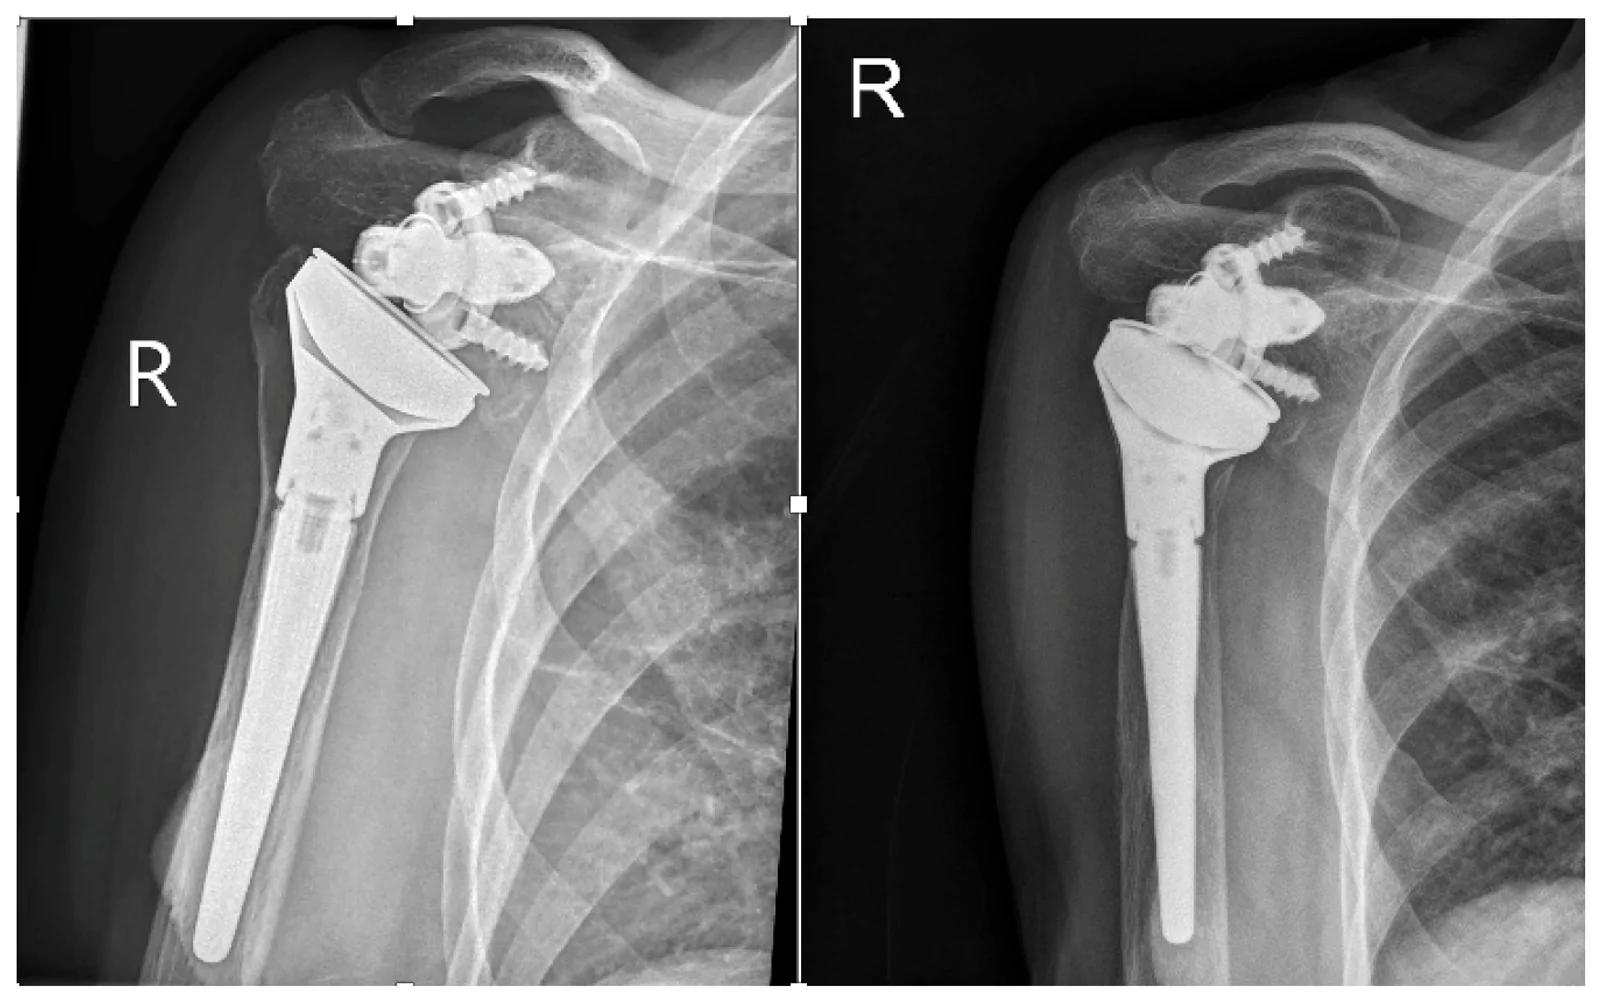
Radiograph of patient with a polyethylene glenosphere taken at 8 and 14 years postoperatively. Note grade 1 notching progressing to grade 2, with progression of stress shielding around humeral component.
